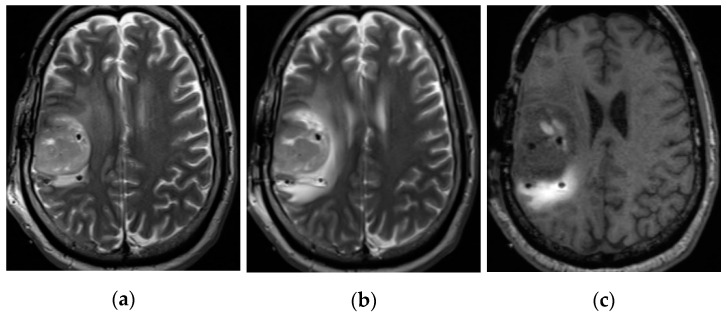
Infusion induces expansion (Axial T2-weighted images, MR1-1 Patient 8). (a) After 2 h of infusion, a small amount of edema (bright regions) is visible near an infusion catheter (black dots), posterior to the tumor in this T2-weighted image. (b) After 24 h of infusion, the size and intensity of the T2-weighted signal in the nearby white matter has increased. (c) T1-weighted imaging after 24 h of infusion shows the distribution of gadolinium tracer).
